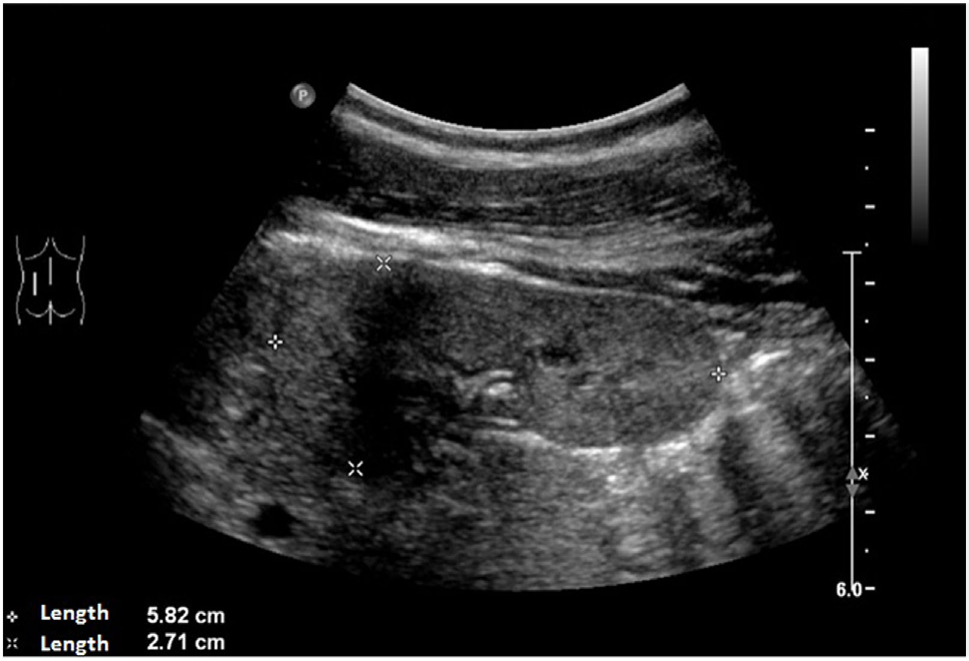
Ultrasound of the left kidney with a length of 5.8 cm (<1st percentile).
